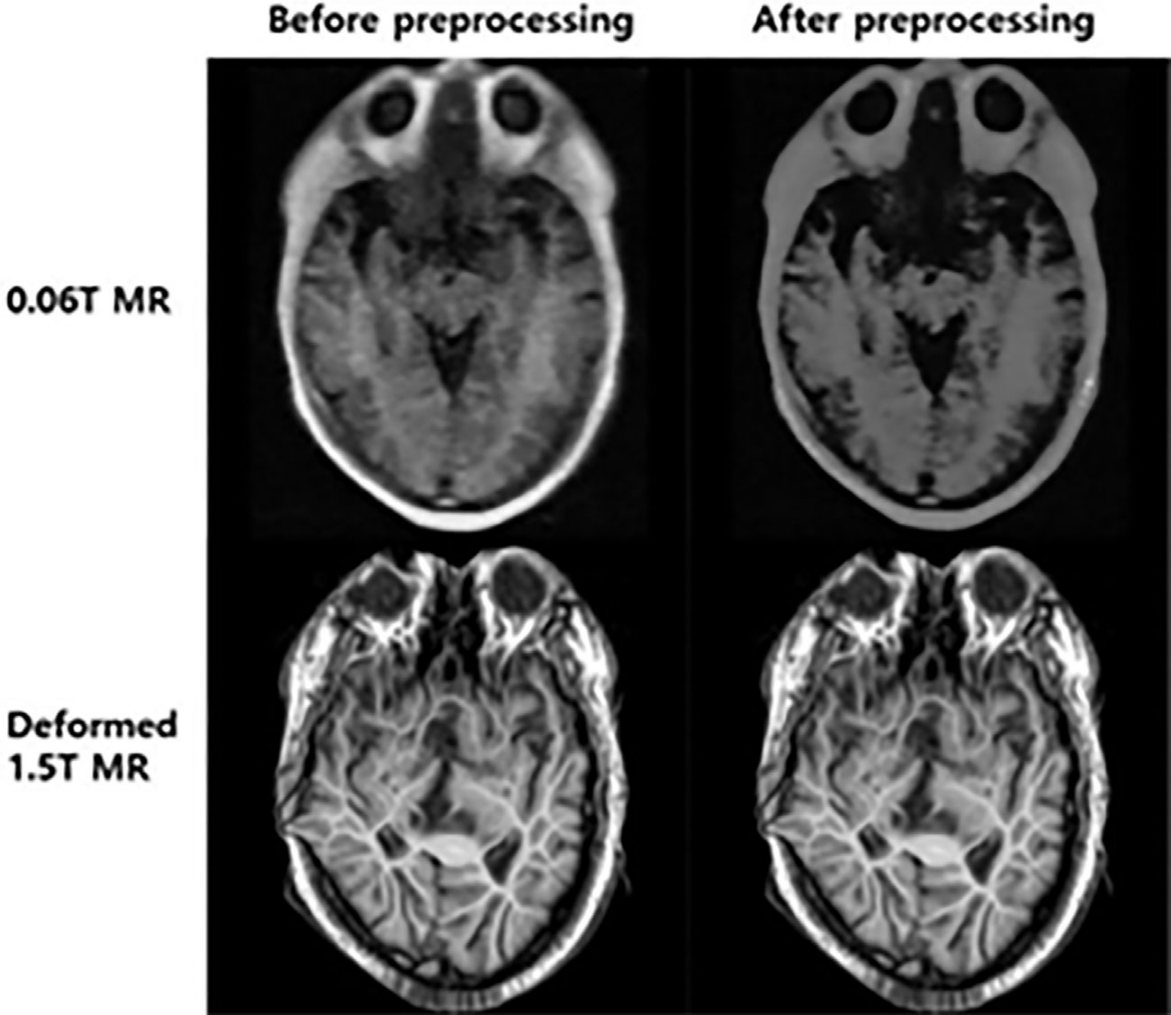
Reduced intensity after preprocessing of MR images (Han’s model).
